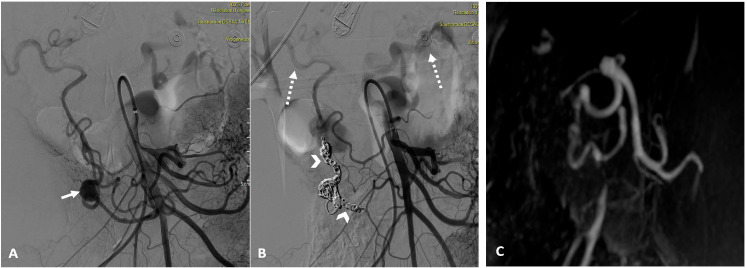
Superior mesenteric arteriography showing an aneurysm of the antero-inferior PDA before (solid white arrow/(A)) and after embolization (B) using the “sandwich” technique and exclusion of inflow and outflow arteries (arrowheads/(B)). Note the reinjection via collaterals into splenic and hepatic arteries (dashed arrows). (C) An MRI angiography in the arterial phase with subtraction in a coronal view with maximum intensity projection reformatting, confirming the total exclusion of the aneurysmal sac 1 month after embolization.
